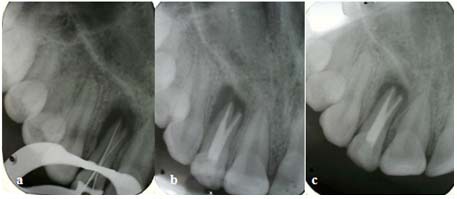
a: Working length determination X-ray b: Post obturation radiograph c: Six months follow-up radiograph
